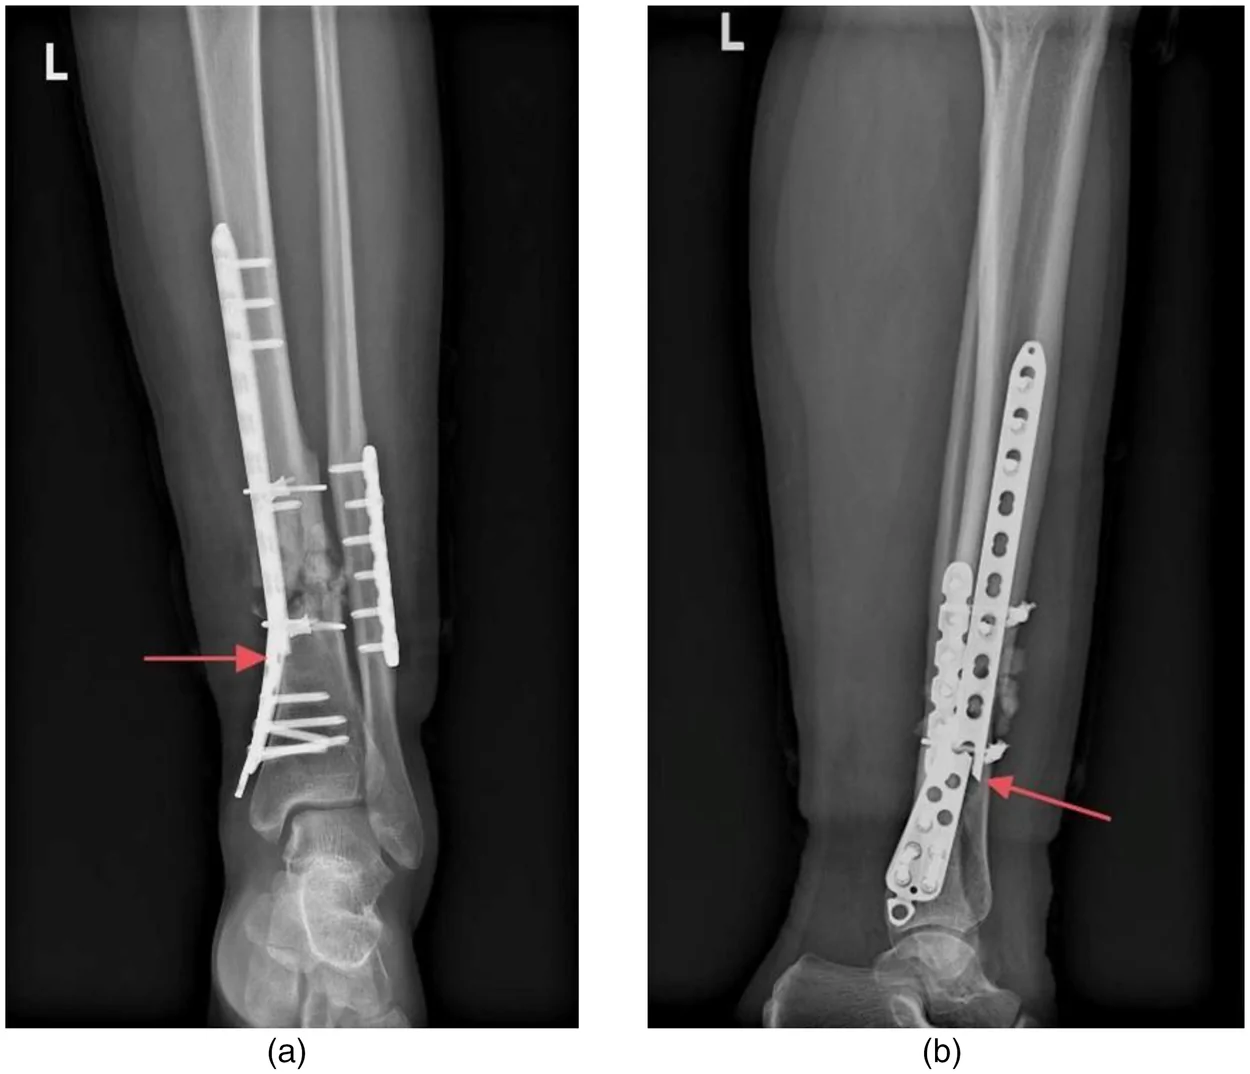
(a,b) on x-ray, plate fracture is observed with nonunion of the fracture site.
